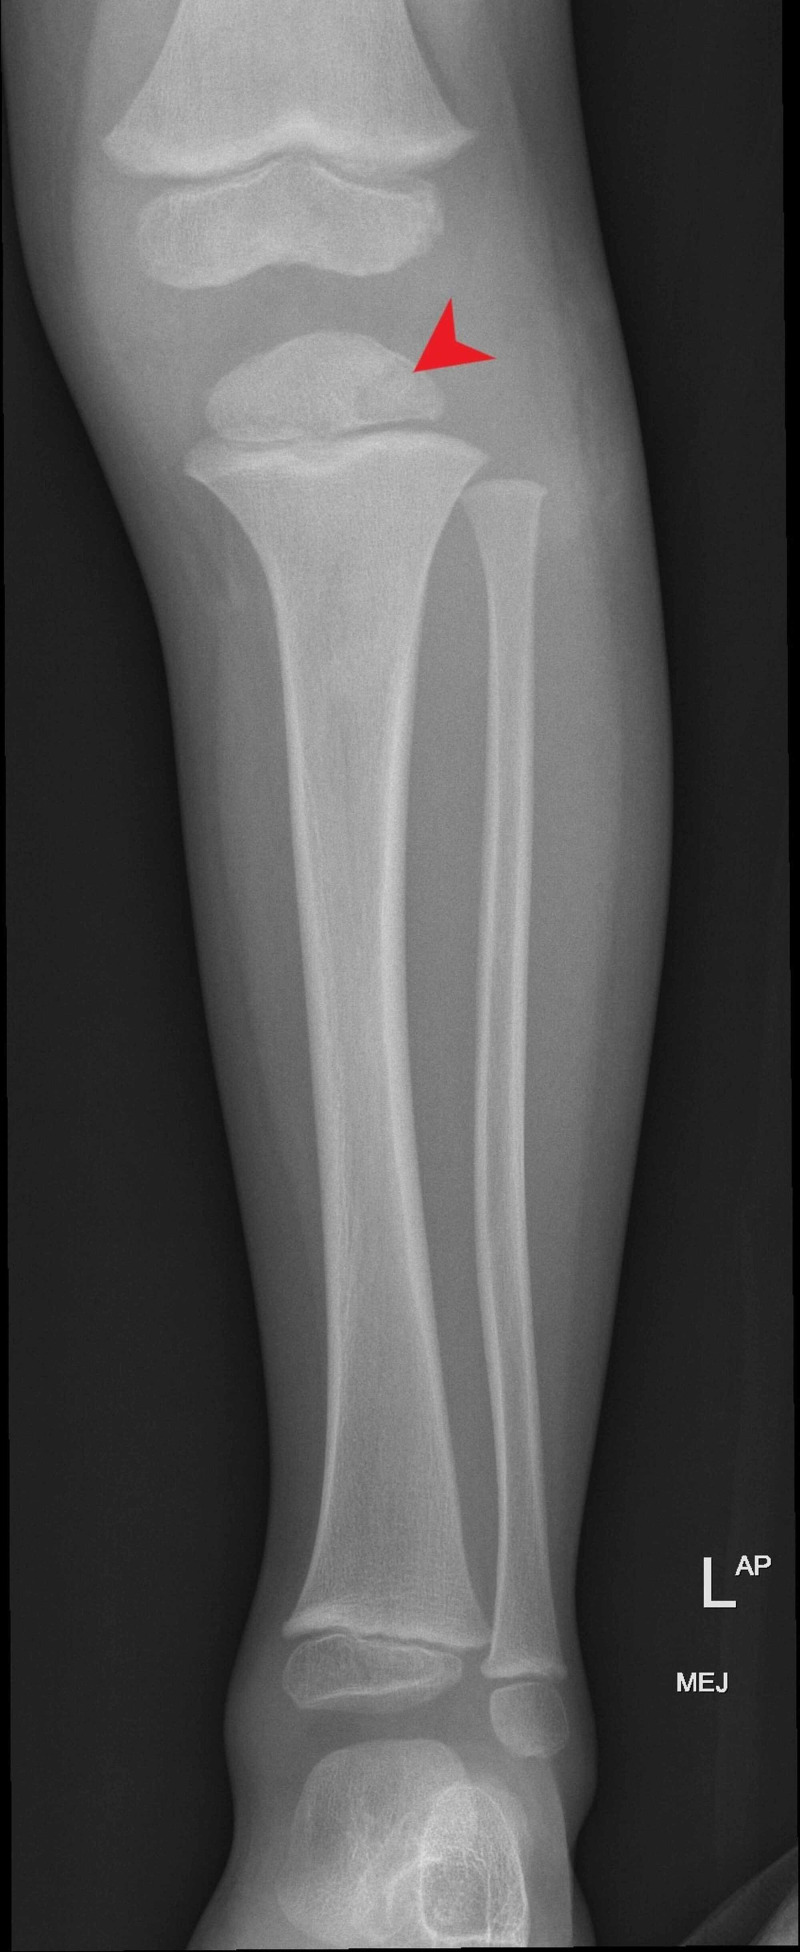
X-ray shows osteolytic lesions of the left proximal tibia.
